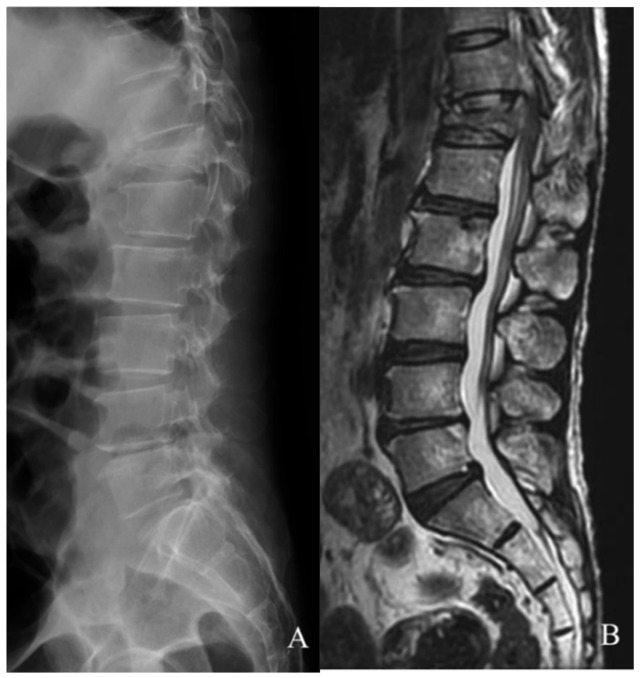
Preoperative imaging (A,B). A burst fracture at T12 resulted in a pan-shaped fragment compressing the conus medullaris that was revealed via X-ray (A) and T2-weighted MRI (B), respectively.
